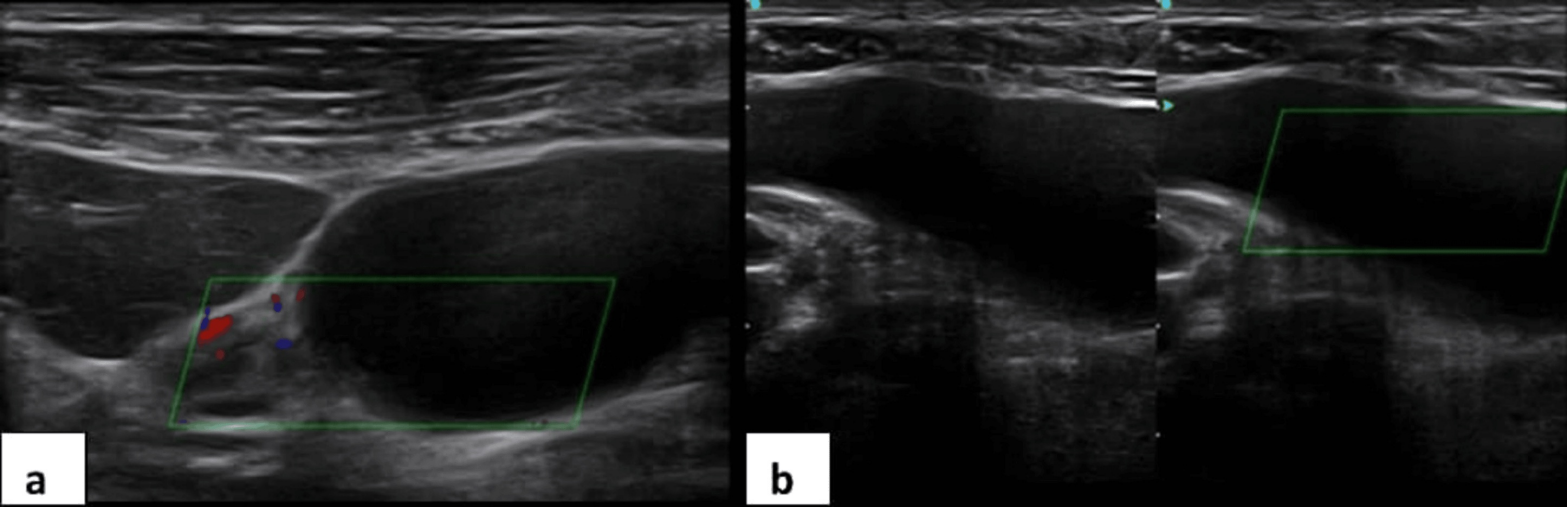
Ultrasound images showing an epigastric cyst later identified as an ectopic gallbladder Ultrasound images showing an epigastric cyst with no color flow located medial to the hepatic lobe, which was later identified as ectopic gallbladder, showing part of the body, neck, and cystic duct (a) and body and fundus (b)
